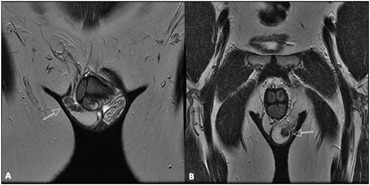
Magnetic resonance imaging (MRI) – Coronal T2 images showing low-sign right (A) and left (B) solid elongated serpiginous lesions in the testicular mediastinum (arrows).
